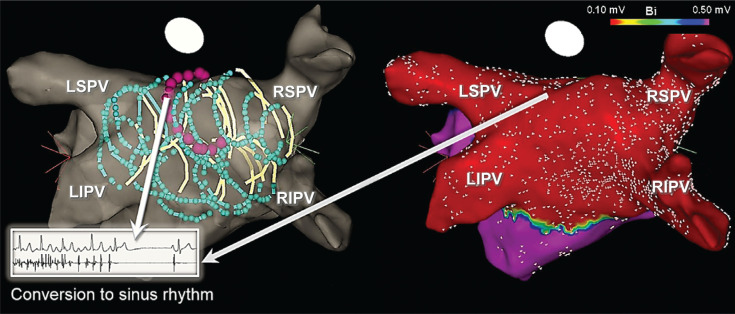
AF termination during cryoballoon PVI + PWI in patients with persAF. Although AF termination and/or conversion to atrial flutters during cryoballoon PVI + PWI is encountered in only 10% to 20% of patients with persAF, when this does occur, it is most commonly observed on the LA roof, as denoted by the magenta-colored application on the ICE-integrated 3D map. ICE: intracardiac echocardiography; LIPV: left inferior PV; LSPV: left superior PV; RIPV: right inferior PV; RSPV: right superior PV.
